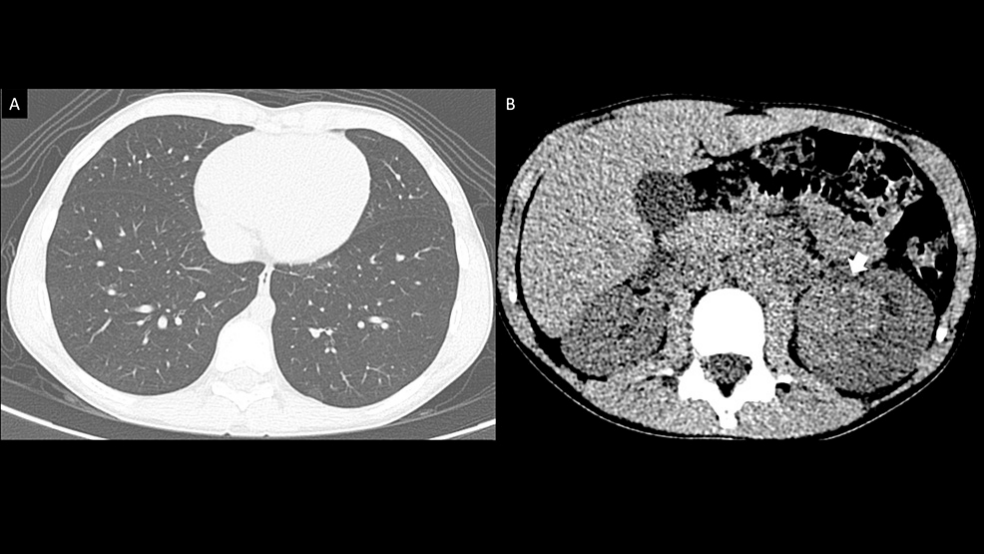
Non-contrast chest CT. Non-contrast chest CT in lung window (A) and soft tissue window (B). Lung parenchyma with no metastasis (A). Hyperdense left renal lesion (arrow in B).
